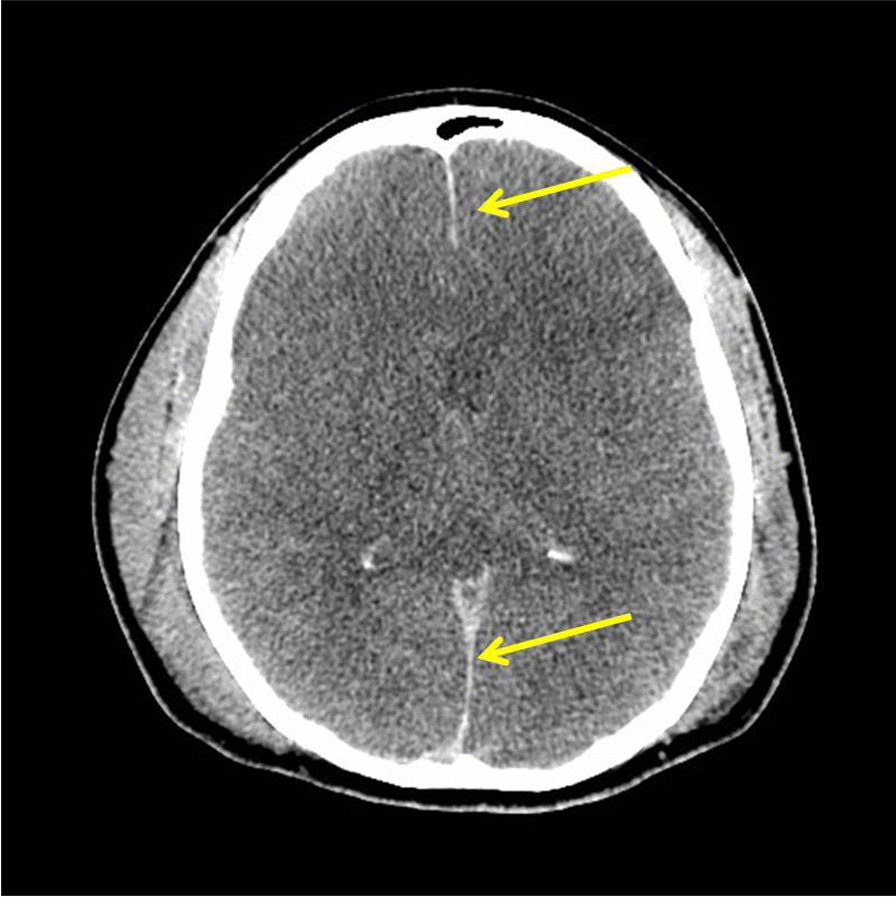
Computerized tomography (CT) head - arrows pointing towards diffuse cerebral edema
